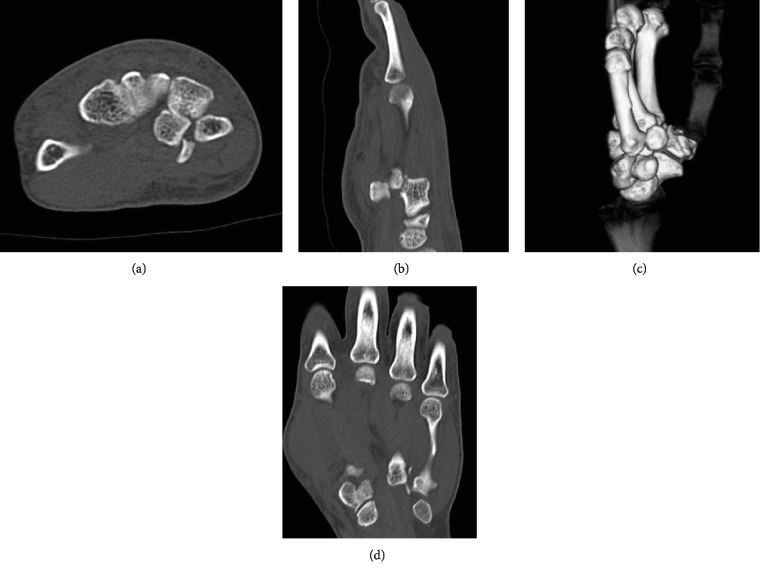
CT scan showing the carpometacarpal bases incarcerated at the fracture gap of the hamate hook at the fracture site. (a) Axial view. (b) Sagittal view. (c) Three-dimensional view of the hand. (d) Coronal view of the CT scan also shows the avulsion fracture between the base of the fourth and fifth metacarpal bones.
